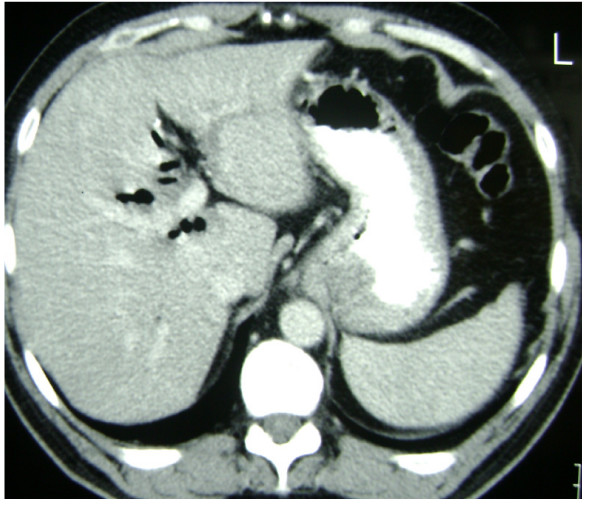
CT appearance. Pneumobilia in CT.
